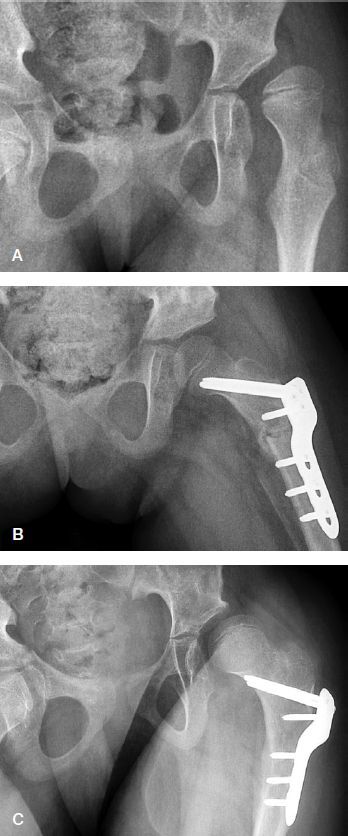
A. Preoperative radiograph of a girl, aged 8.1 years and GMFCS level V, with severe subluxation of her left hip (MP 74%). B. 6 weeks after femoral and pelvic osteotomies of the left hip and bilateral soft tissue releases, showing good femoral head coverage. C. 2.9 years postoperatively (age 11.0 years), showing relapse of subluxation of her left hip (MP 51%).
